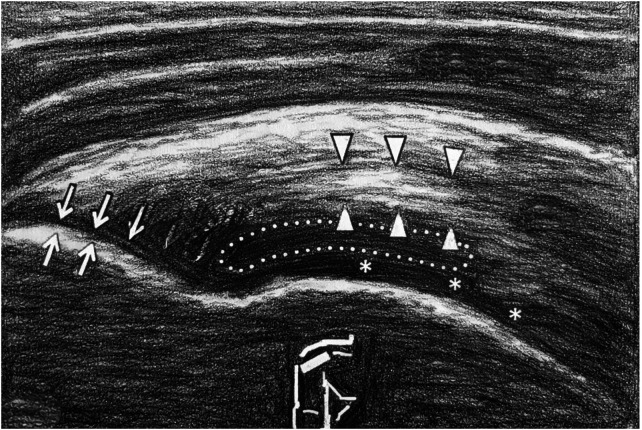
Ultrasound anatomy of the shoulder's anterior approach. ↑: SSP tendon insertion point on the greater tuberosity; ▴: typical hyperechogenic tendon area; *: humeral head cartilage.
